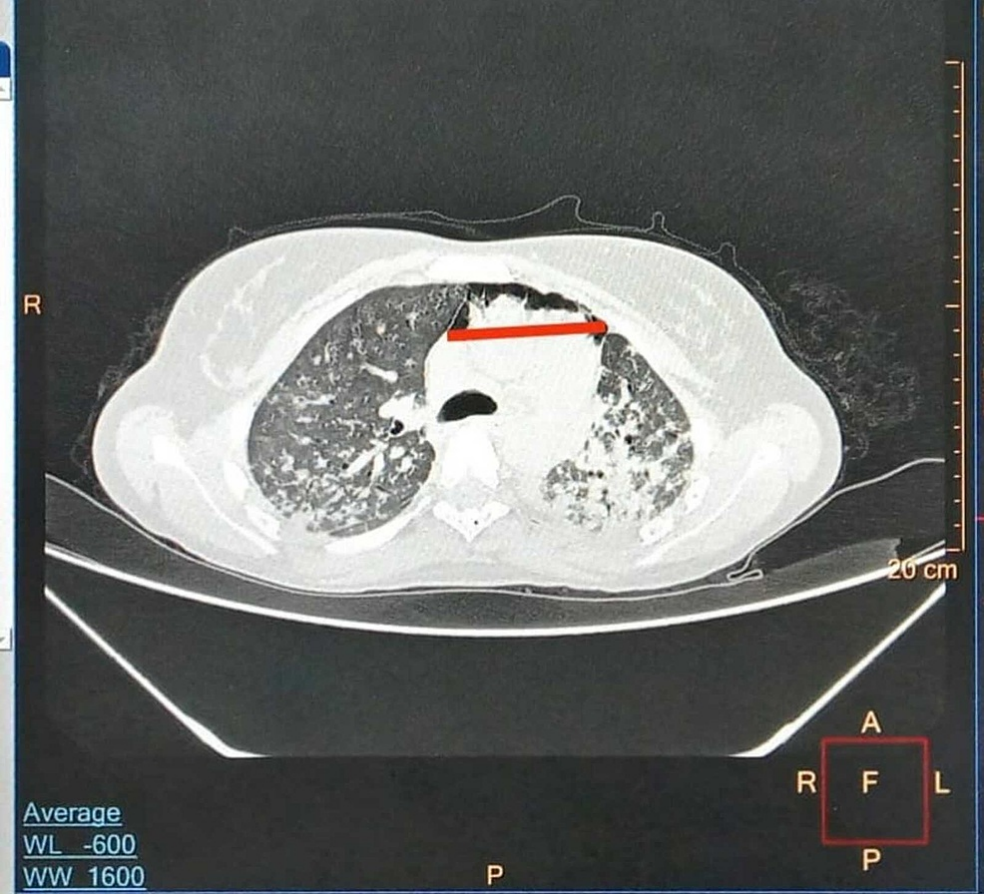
High-resolution computed tomography of the thorax High-resolution computed tomography image of the chest showing extensive ground glass opacity with pneumomediastinum of size 8.5 mm.
